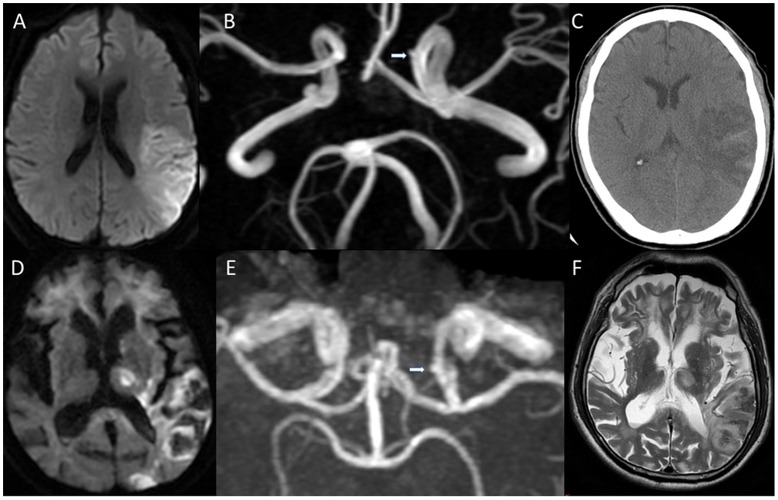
Representative images of patients with intracranial aneurysm with a complicated post-r-tPA intracranial hemorrhage. (A-C) The 72-year-old man had acute ischemic stroke and had received r-tPA within 3 h after the onset of stroke. Follow-up diffusion-weighted imaging (A) (2 h after r-tPA) revealed acute ischemic stroke over the left middle cerebral artery territory. (B) Magnetic resonance angiography revealed a small (2 mm) saccular form of intracranial aneurysm (arrow) over the left internal carotid artery (Source images of magnetic resonance angiography is available in the S1 Fig). (C) Follow-up computed tomography performed 2 days after r-tPA demonstrated petechial hemorrhage in the infarcted margin. (D-F) The 77-year-old woman had acute ischemic stroke and had received r-tPA within 3 h after the onset of stroke. Follow-up diffusion-weighted imaging (D) (2 days after r-tPA) revealed acute ischemic stroke over the left middle cerebral artery territory. (E) Magnetic resonance angiography revealed a small (2.5 mm) saccular form of intracranial aneurysm (arrow) over the left internal carotid artery (Source images of magnetic resonance angiography is available in the S2 Fig). (F) T2-weighted image demonstrated petechial hemorrhage over the infarcted areas without mass effect.
